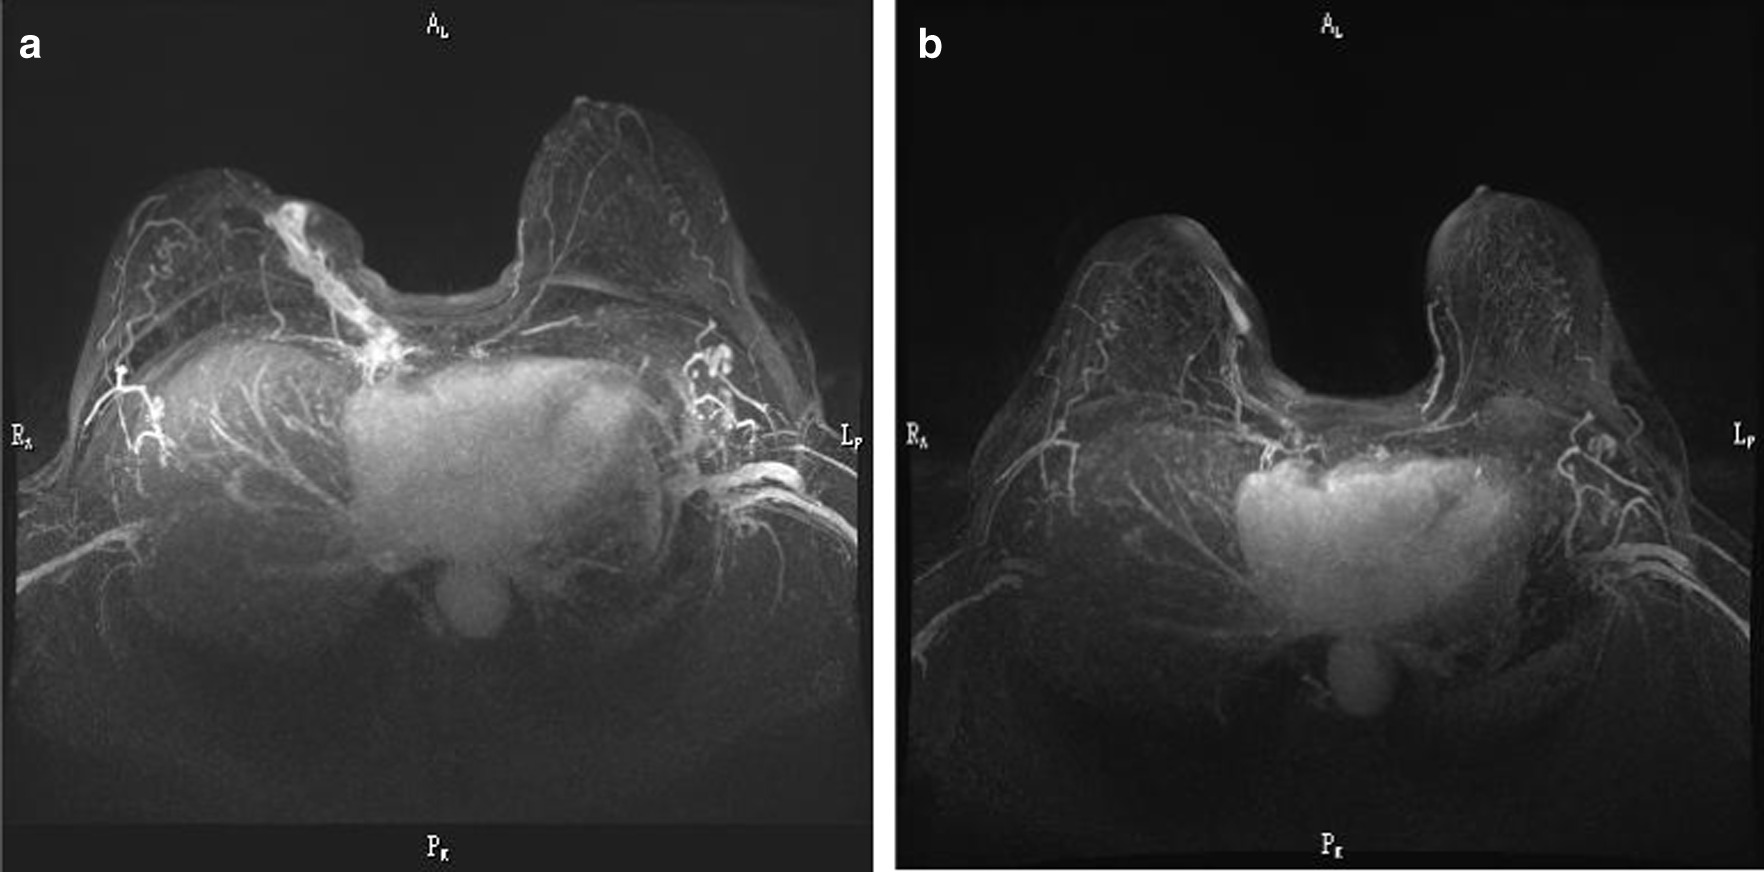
Dynamic subtraction magnetic resonance imaging of the breast. a Before treatment: formation of a fistula between the parasternal area and skin of the right breast. b After completion of antituberculosis treatment
